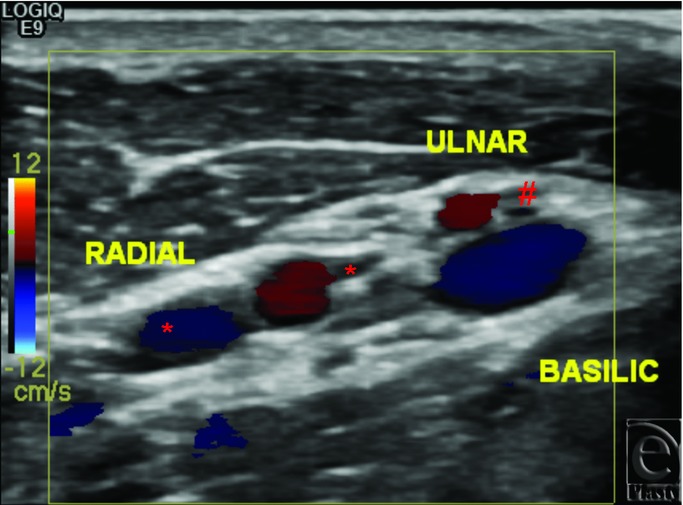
Vascular ultrasonography. Preoperative vascular ultrasonography at the proximal right upper arm of subject 1 demonstrates high arterial bifurcation of brachial into radial and ulnar arteries. Also seen are 2 radial veins (*), one ulnar vein (#), and the basilic vein.
